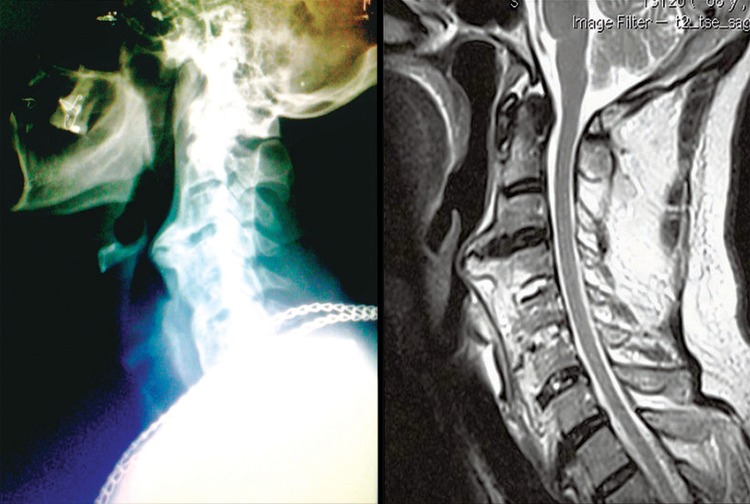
Lateral cervical X-ray and T2-weighted sagittal magnetic resonance. We observed an extensive calcification in front of vertebral bodies of C2 to C6 in topography of anterior longitudinal ligament. Esophagus and airways present a posterior compromising, which determine a stenosed segment that difficult orotracheal intubation at time of surgical procedure
